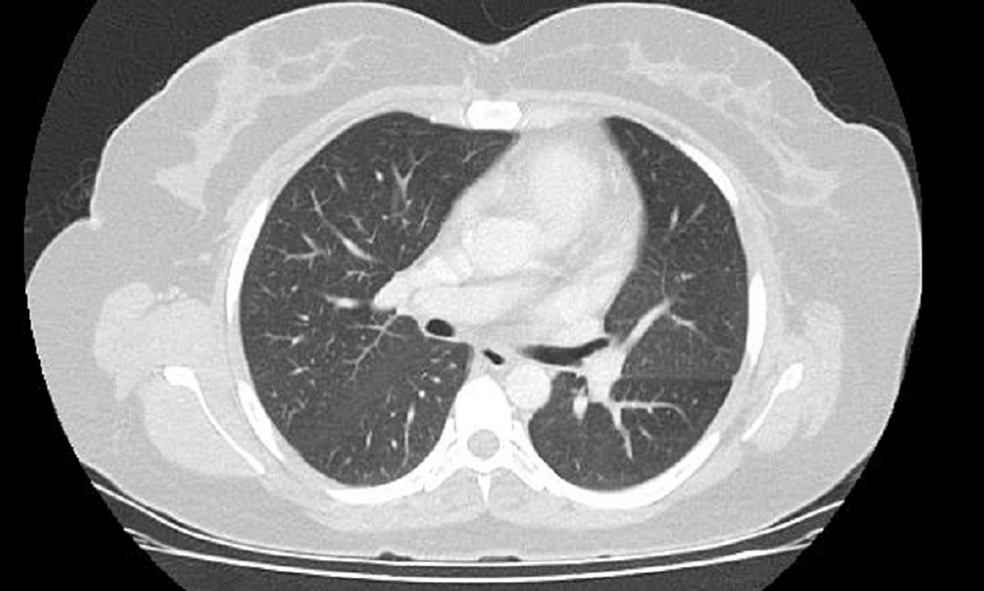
Recent CT scan of the chest showing response to alectinib. CT: computed tomography
